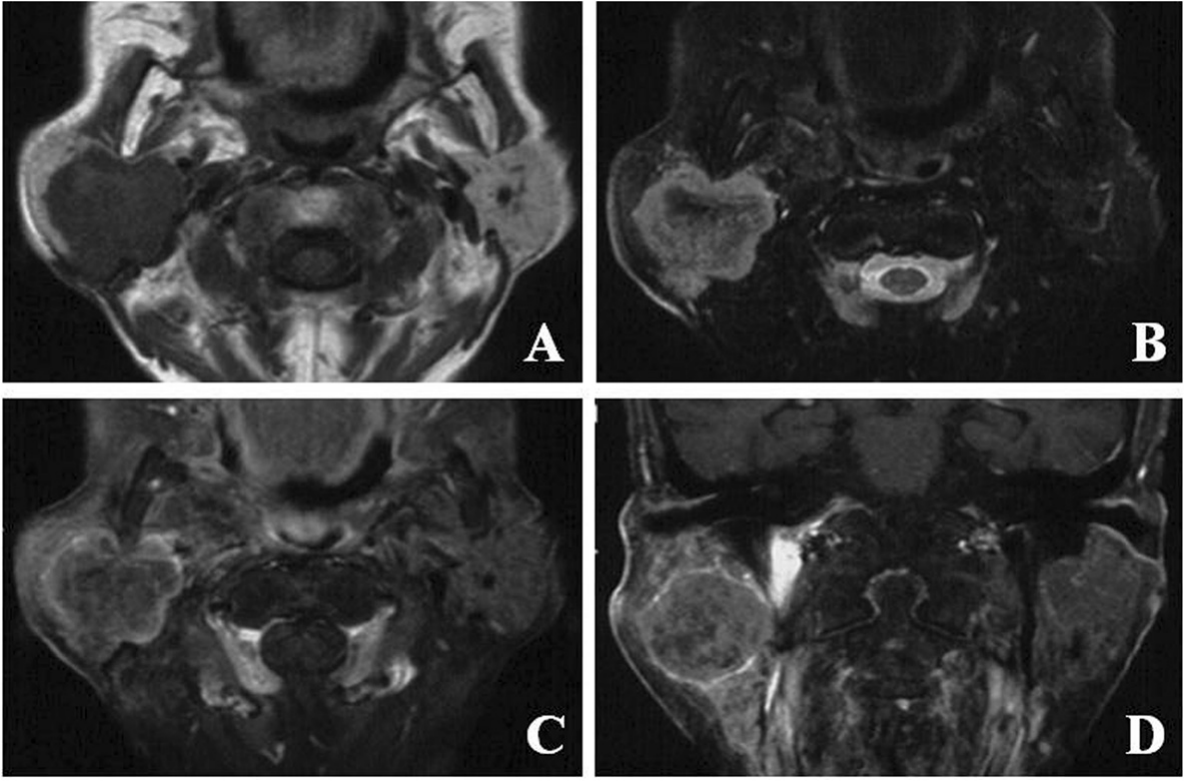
Magnetic resonance imaging scans. Magnetic resonance imaging shows this lesion to be clearly circumscribed, with (A) homogeneous hypo-iso signal intensity on T1-weighted images and (B) heterogeneous high signal intensity on T2-weighted images. (C) Axial and (D) coronal gadolinium-enhanced T1-weighted images show the presence of a circumscribed and heterogeneously enhanced tumor.
